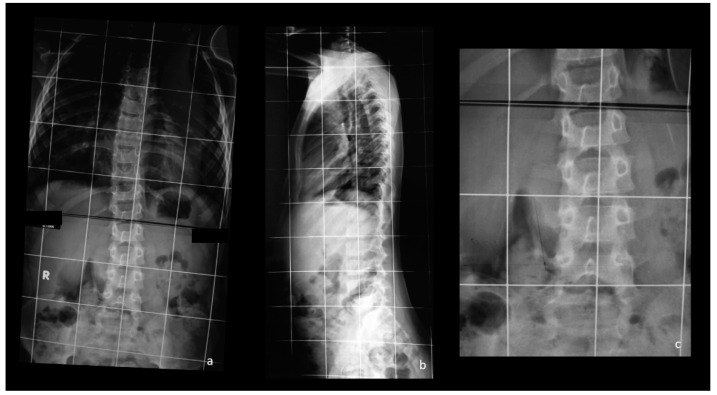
Anteroposterior (a) and latero-lateral (b) views of a lumbar dystrophic early onset scoliosis. Note the dysplastic pedicles of the lumbar spine, the intervertebral foraminal widening in (b). In (c), a detail of the anteroposterior view. Note the apparent overlapping of bone between the vertebrae on the concave side, lately confirmed by MRI to be paravertebral neurofibromas.
